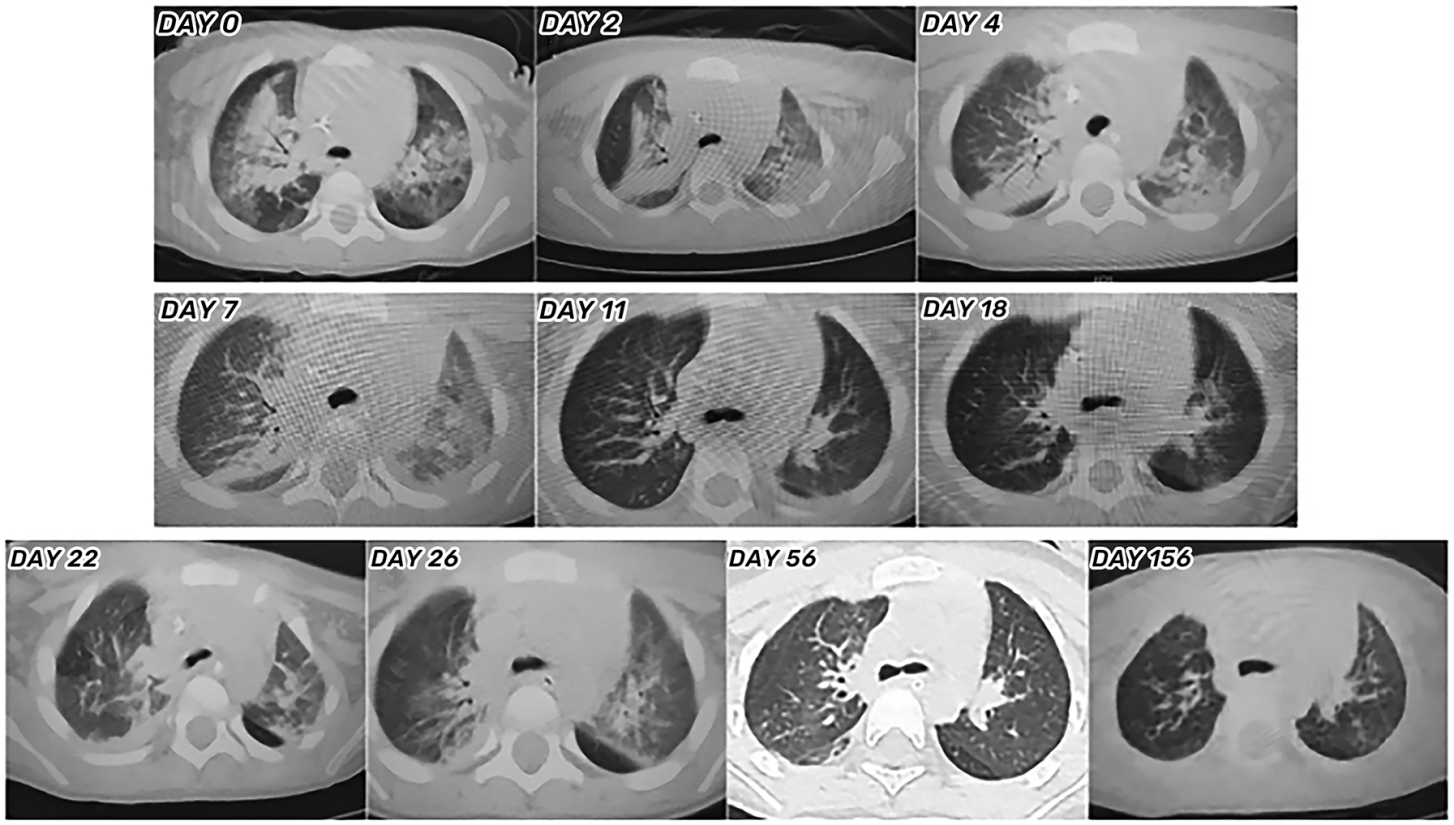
Lung computed tomography scans of the toddler during hospitalization and follow-up.
